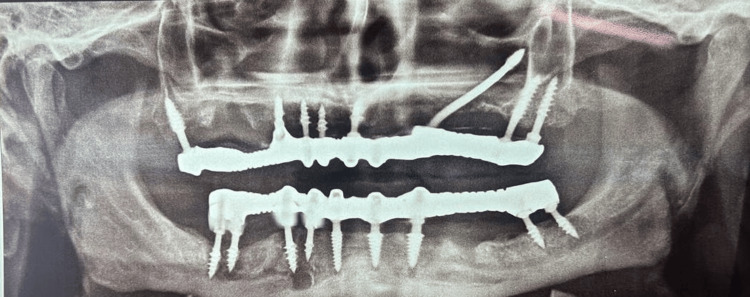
Postoperative OPG of the patient OPG: Orthopantomogram
